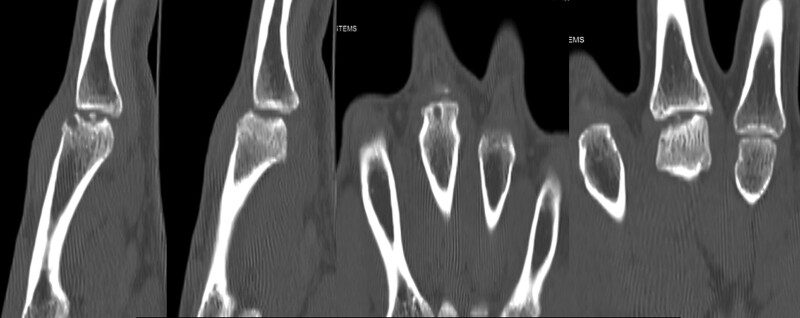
Computed tomography scans of the right hand demonstrating cystic change, fragmentation of the 3rd metacarpal head.
